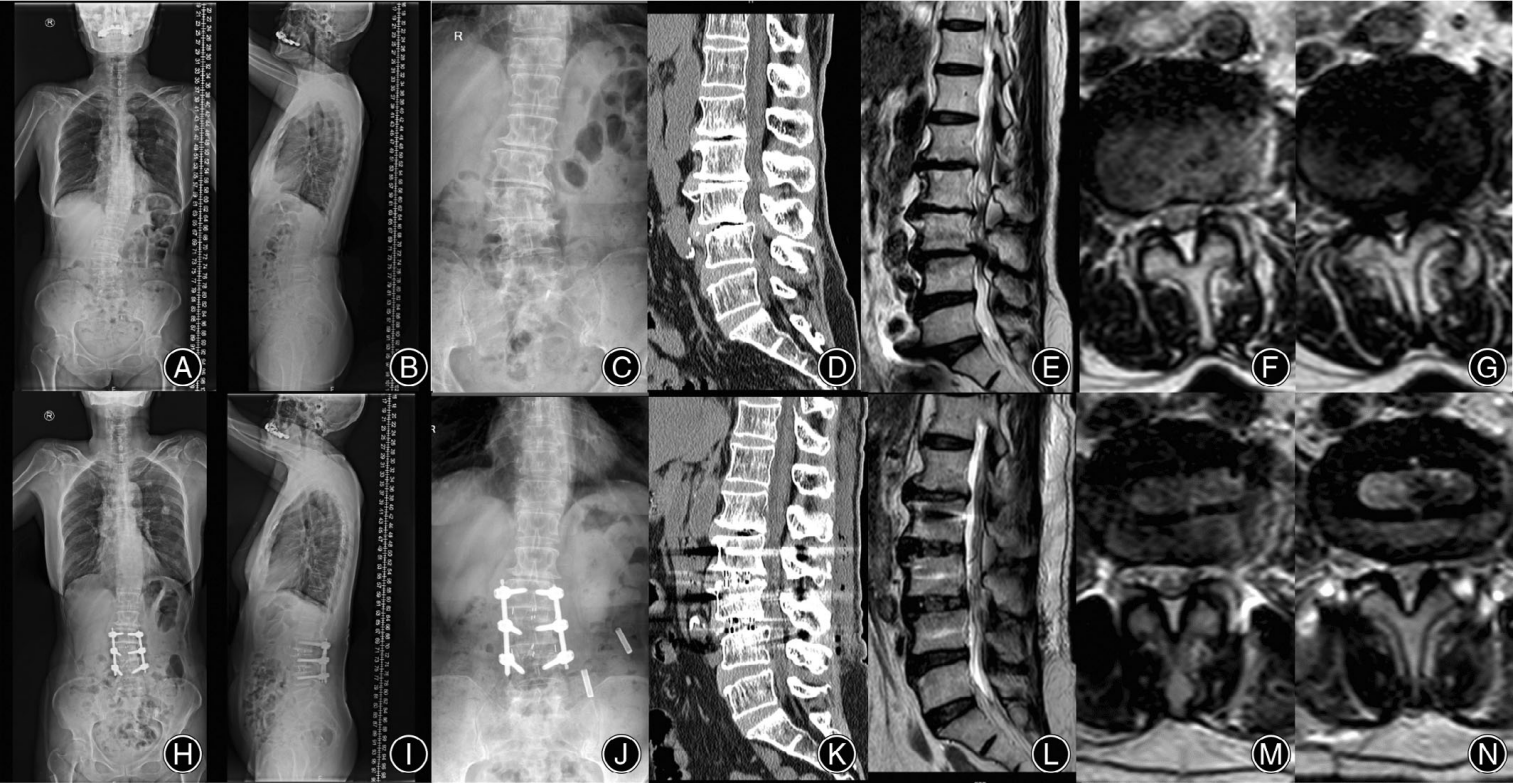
X‐rays showed scoliosis of the lumbar spine, lateral slip of L_3_, and lateral bone bridge at L_2/3_ level (A–C). CT showed L_3_ spondylolisthesis and stenosis at L_3/4_ intervertebral space (D). MRI showed severe stenosis at L_3/4_ with Schizas grade D (E‐G). Postoperative X‐rays showed that lumbar scoliosis was improved (H–J). CT showed that the lumbar spondylolisthesis was improved and the height of the intervertebral space was restored (K). MRI showed that the spinal canal stenosis at L_3/4_ was improved to Schizas grade B (L–N).
